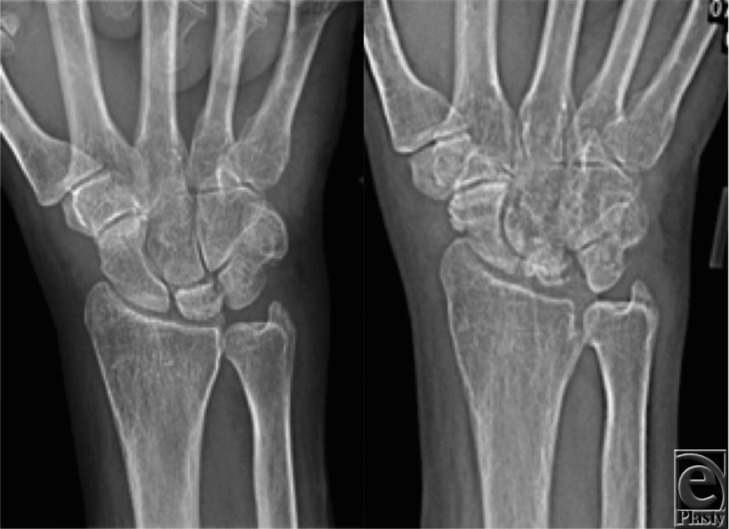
Preoperative posteroanterior radiographs showing progressive fragmentation and collapse of the lunate, as well as subsequent pathologic fracture of the scaphoid.
